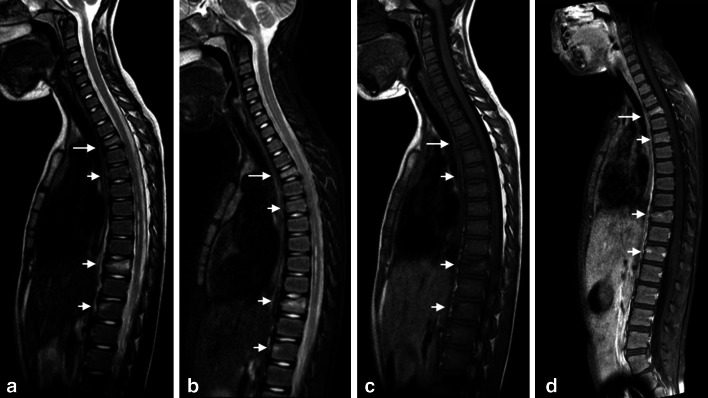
A 7-year-old girl with Langerhans cell histiocytosis (LCH) presenting with back pain. Sagittal T2-weighted (a), STIR (b), T1-weighted (c), and contrast-enhanced fat saturated T1-weighted (d) images of the spine showing involvement of multiple vertebral bodies (*small arrows*) as well as a “vertebra plana” at the level of Th3 (*large arrow*). The lesions are best seen on the precontrast T1-weighted and T2-weighted images
